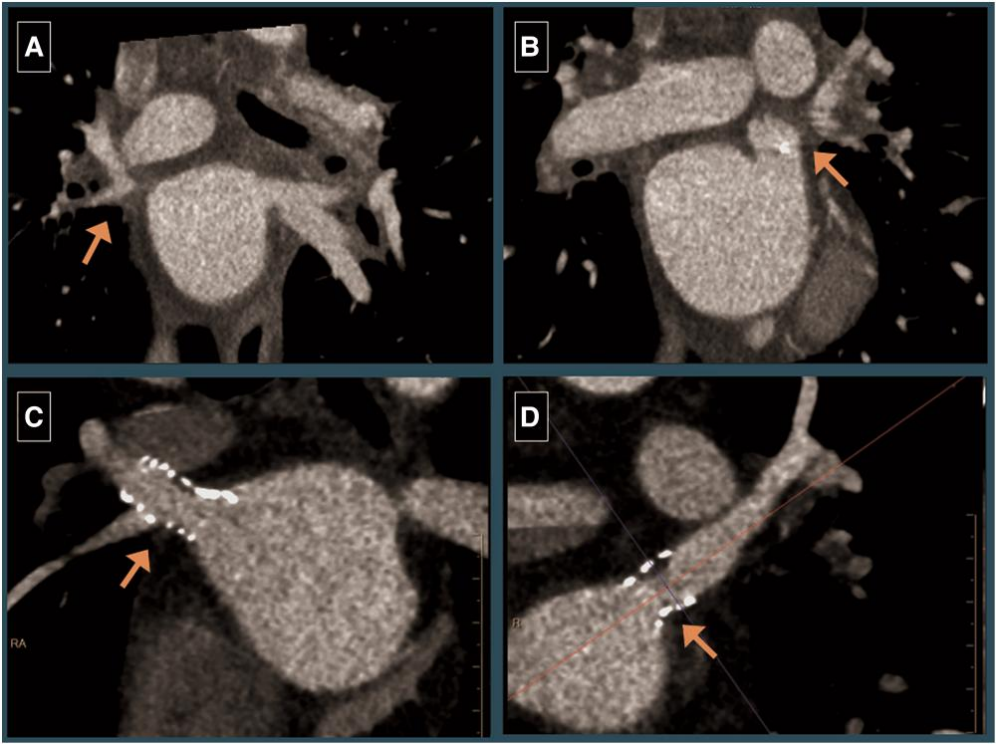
(Left to right). Baseline CTA showing severe stenosis of the right (*A*, arrow) and left (*B*, arrow) superior pulmonary veins. Surveillance CTA 3 months post-procedure showing patient stents in the right (*C*, arrow) and left (*D*, arrow) pulmonary veins.
